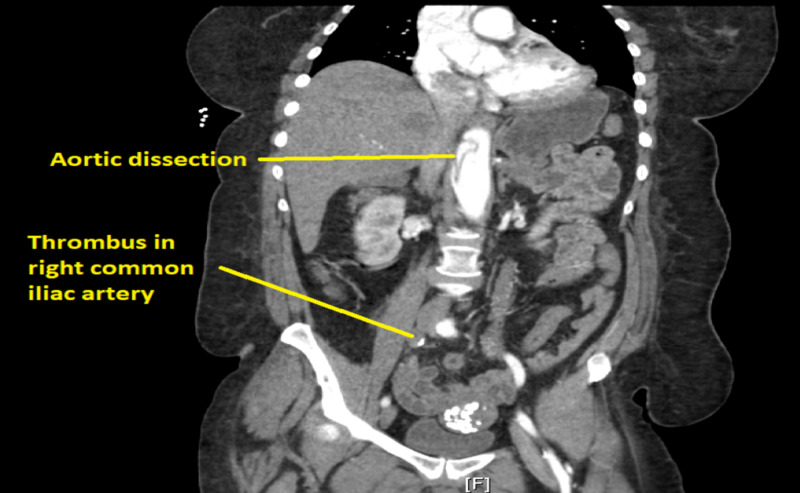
CT scan showing thrombus occluding right common iliac artery and aortic dissection.
